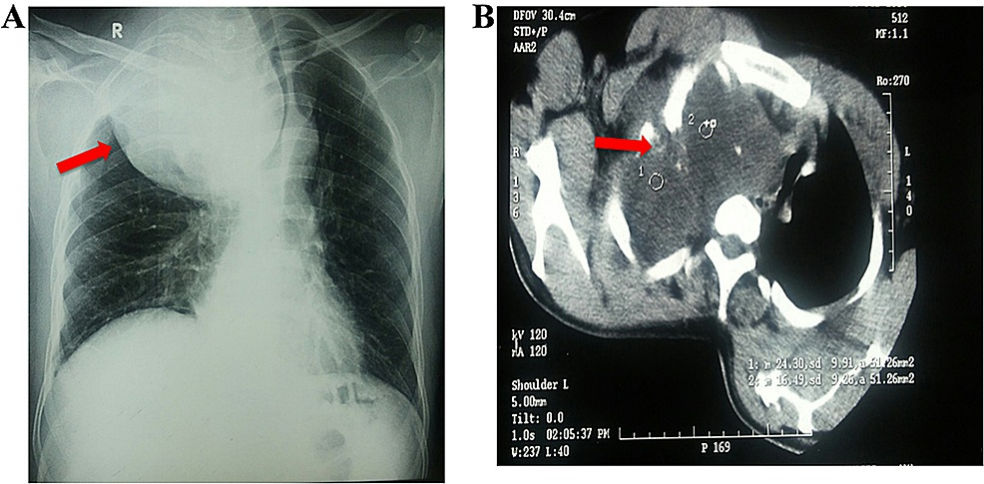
(A) Chest X-ray; (B) contrast-enhanced CT scan. Red arrows represent chondroid hamartoma in radiological figures. CT: computed tomography
